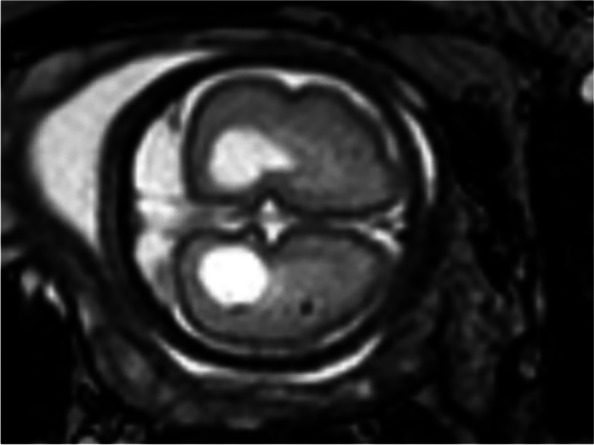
Prenatal fetal MRI showed a short T2 signal in the white matter of the right parietal lobe of the fetus
